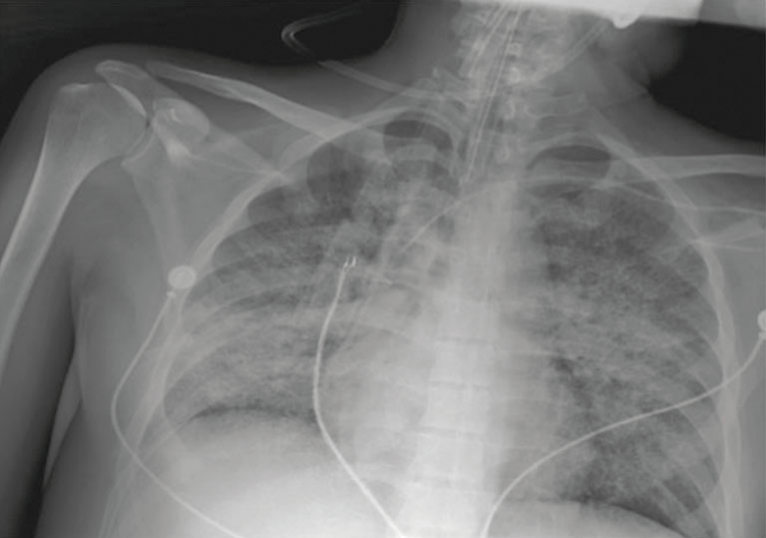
Chest X-ray with an extensive nodular pattern in both lung fields.
